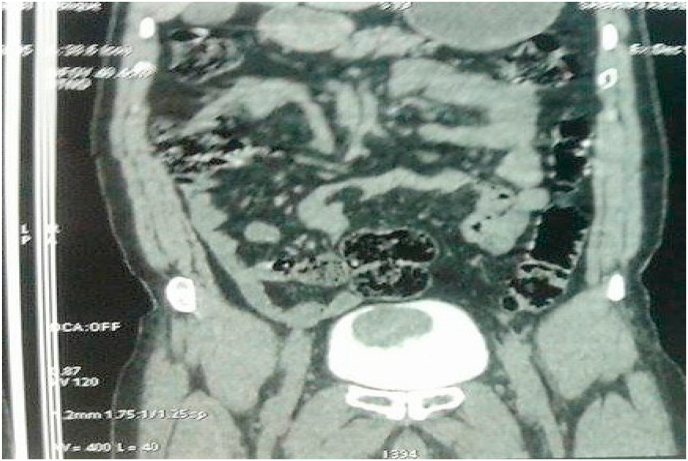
Abdomino-pelvic CT scan revealed wall thickening, budding from the anterior wall of the bladder.
